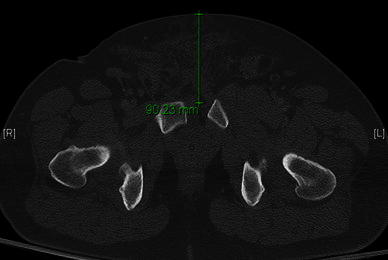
Axial CT image of an obese patient who had a complication following vertical midline skin incision. The distance between the pubic symphysis and skin was measured to be 9 cm
